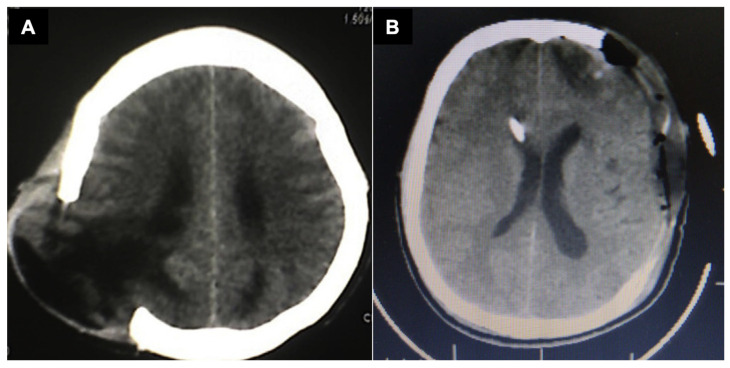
Panel (A) shows a CT scan demonstrating inadequate decompression with associated brain herniation (“fungus cerebri”) and established cerebral infarction due to insufficient cranial expansion. Limited craniectomies fail to achieve meaningful intracranial volume augmentation and are associated with a higher risk of secondary injury and poor neurological recovery in severe traumatic brain injury. Common complications include cortical infarction, venous congestion or bleeding, external herniation (“fungus cerebri”), and subdural hygromas. Panel (B) shows a CT scan demonstrating a well-performed decompressive craniectomy. There is a large frontal-temporal-parietal bone flap removal (anteroposterior diameter ≥12 cm) with adequate basal temporal decompression.
